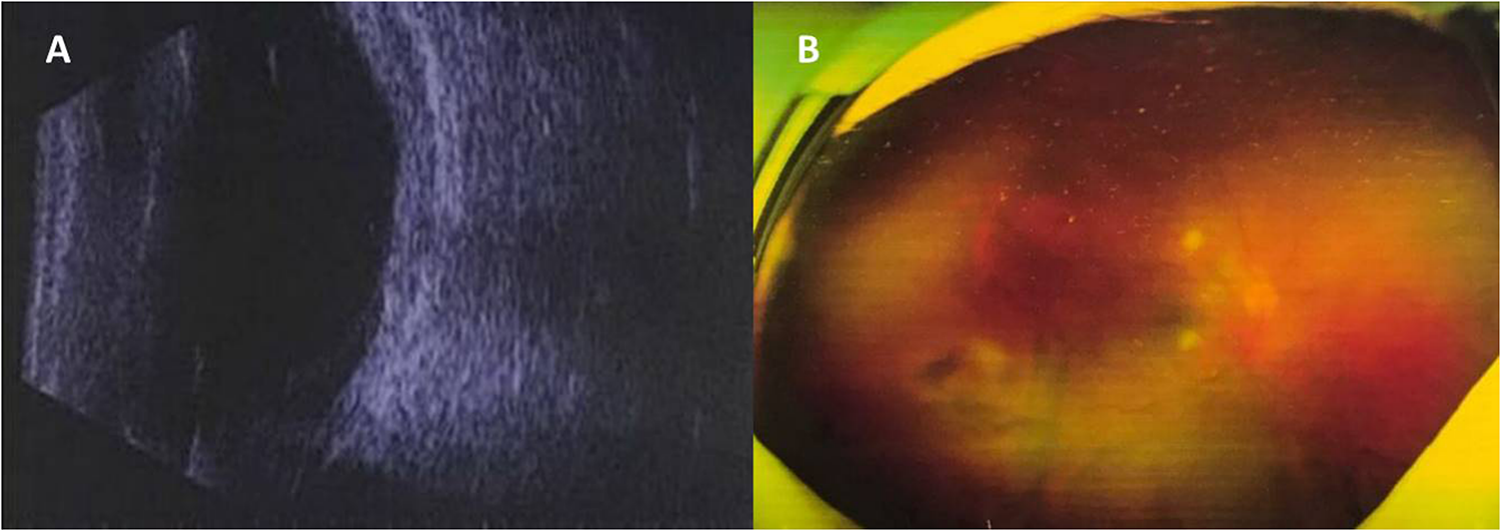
Fundus findings after surgery: case 1. (A) B-ultrasound scan showing slight turbidity of the vitreous body and vitreous organization after surgery. (B) Vitreous hemorrhage was partially absorbed and the optic nerve head could be seen faintly after surgery.
